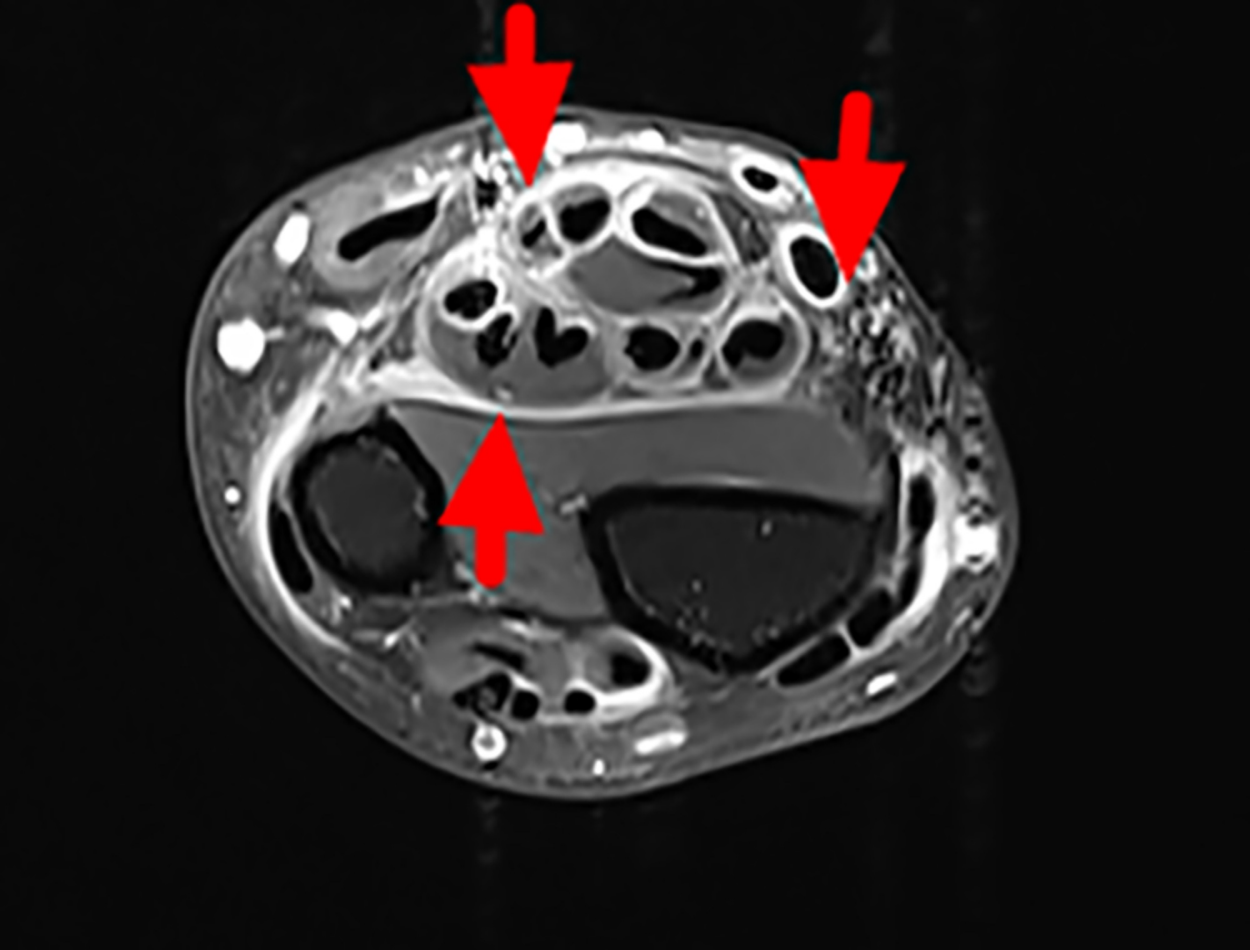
MR scan of the left wrist Axial enhanced T1-weighted fat-suppressed MR images showing thickening and enhancement of the fascia and tenosynovitis of flexor and extensor tendons. MR, magnetic resonance
